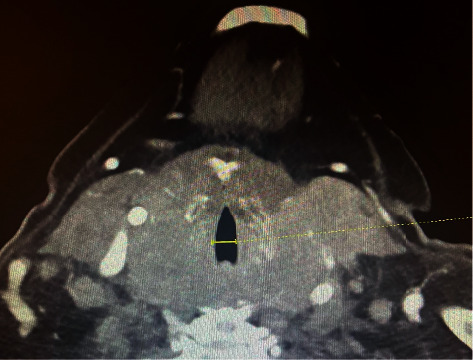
CT neck scan with contrast showed a markedly enlarged bilateral thyroid gland measured approximately 7.8 × 7.2 cm, with marked transverse narrowing of the trachea at the level of the thyroid gland to approximately 8.6 mm (solid yellow line).
